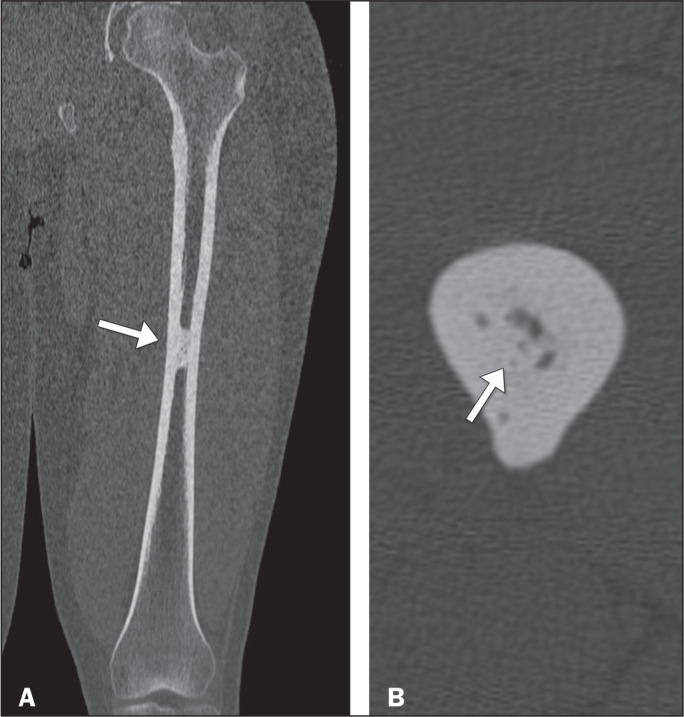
Coronal (A) and axial (B) CT scans of the lower limbs of a
